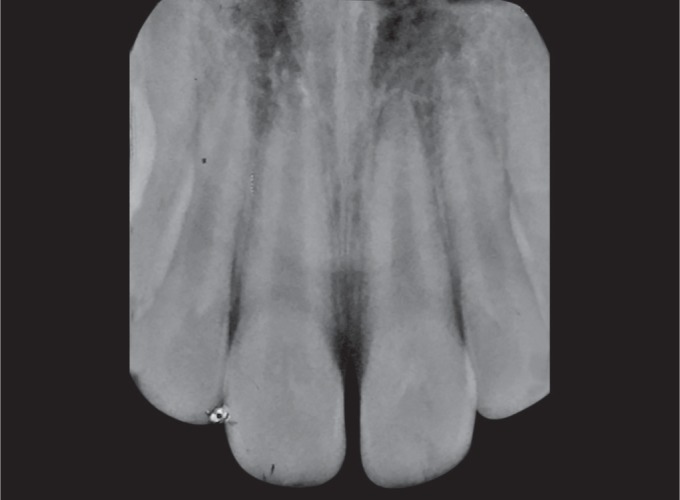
Periapical radiograph taken after stabilizing the coronal fragment
